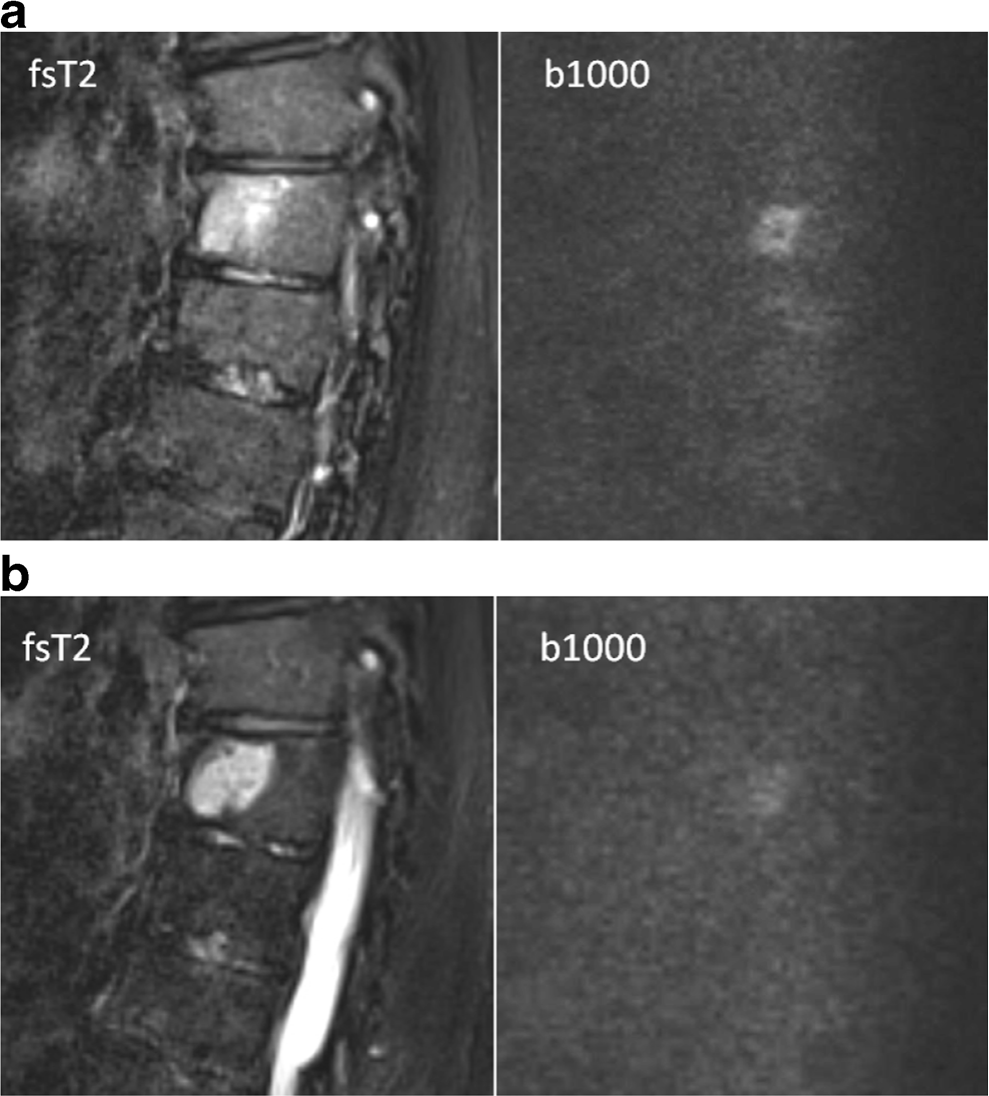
T2-weighted (*left*) images of a hyperintense focal lesion (*white arrow*) a in a thoracic vertebra of a newly diagnosed myeloma patient with the corresponding b1000 image (*right*), showing the focal lesion together with a slightly diffusely increased signal intensity in the all vertebral bodies. b After systemic treatment and local irradiation on the focal lesion the signal intensity of the focal lesion increases on the T2-weighted image, indicating response and tumour necrosis, with residual hyperintensity on the b1000 image (*right*), due to the large water amount after necrosis: T2-shine-through effect
